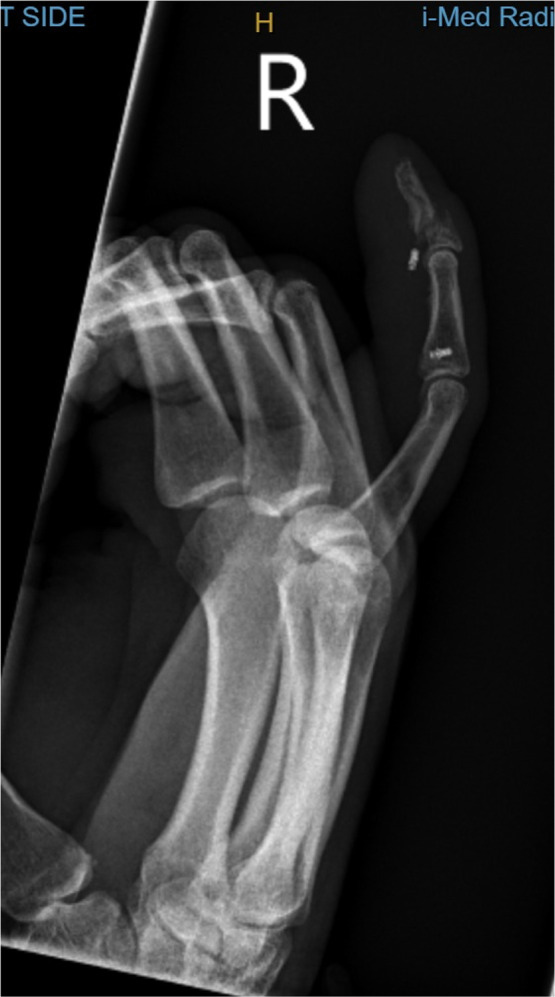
Lateral X-Ray of right hand (Case 1).
